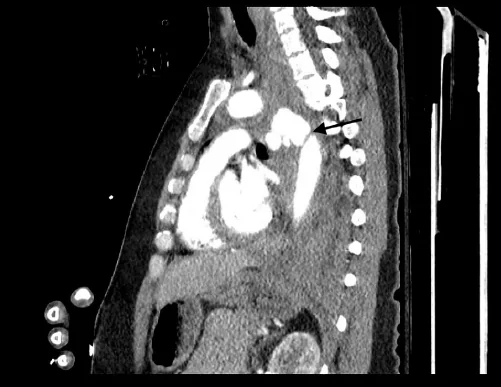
Computed tomography of the chest with contrast in sagittal view demonstrating the full extent of the aortic transection (arrow). Findings are consistent with a grade four blunt thoracic aortic injury. The transection extends into the proximal portion of the descending aorta.
